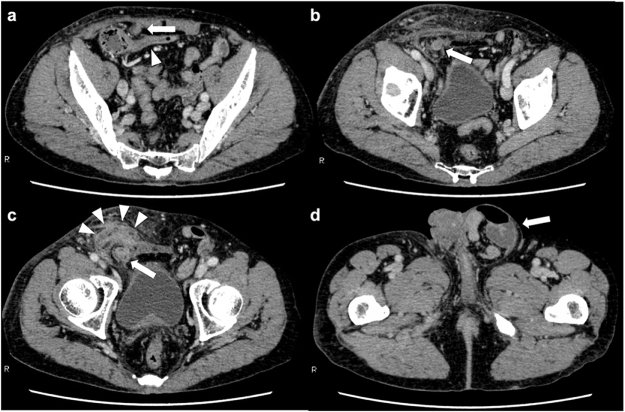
Axial computed tomography scan of the abdomen before the first surgery. (a and b) Right Amyand’s hernia containing the appendix (arrows) contiguous with the terminal ileum (arrowhead). (c) Right inguinal hernia with multiple abscesses (arrowheads). (d) Left inguinal hernia containing a part of small intestine (arrow).
